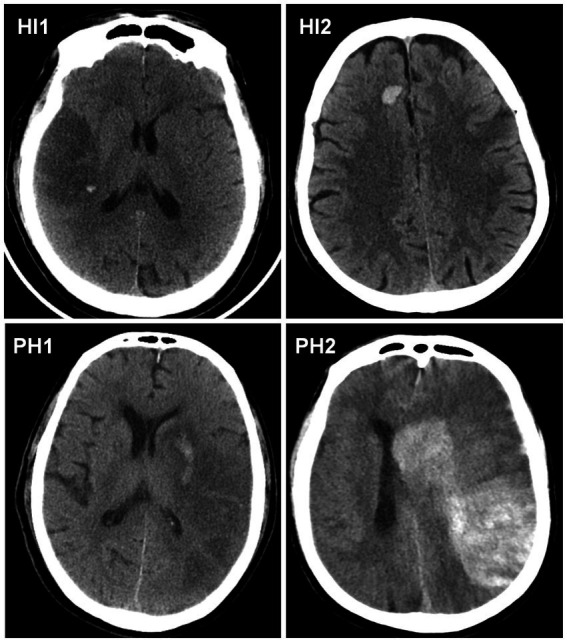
CT scan examples of hemorrhagic infarction type 1 (HI1), HI2, parenchymal hematoma type 1 (PH1) and PH2, according to the European Cooperative Acute Stroke Study criteria. HI1, hemorrhagic infarction type 1; HI2, hemorrhagic infarction type 2; PH1, parenchymal hematoma type 1; PH2, parenchymal hematoma type 2.
